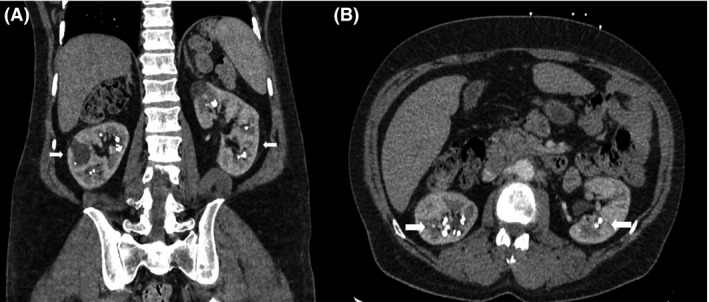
CT scan of the abdomen, transverse, and coronal views, demonstrating bilateral medullary nephrocalcinosis.
